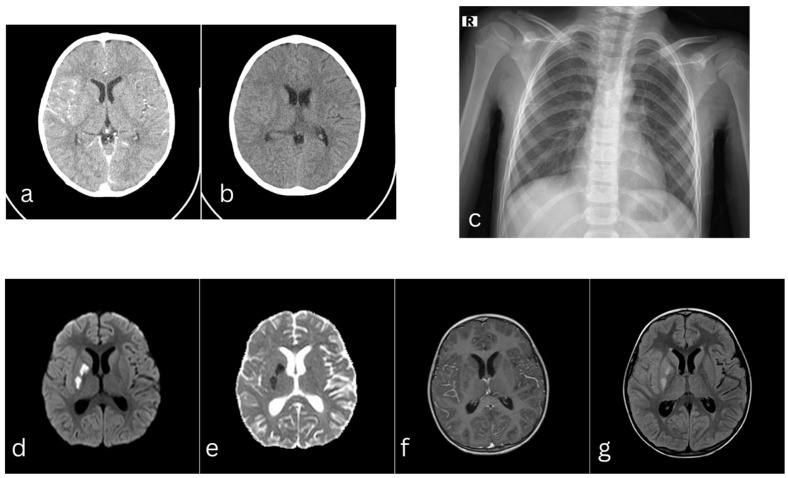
Brain CT scan with contrast (a), and non-contrast (b) on the day of admission, showing mild dilation of the ventricular system without intraventricular pathological content, vascular hypercongestion along the right temporal cerebral gyruses. Chest X-ray (c) shows no significant abnormalities. Brain MRI on the fifth day of admission, DWI/ADC axial scans (d,e), depict focal lesions with restricted diffusion in the right nucleocapsular region consistent with ischemic lesions. T1 axial scan with contrast (f) demonstrated absence of enhancement, and FLAIR axial scan (g) hyperintensity in the right nucleocapsular lesions.
